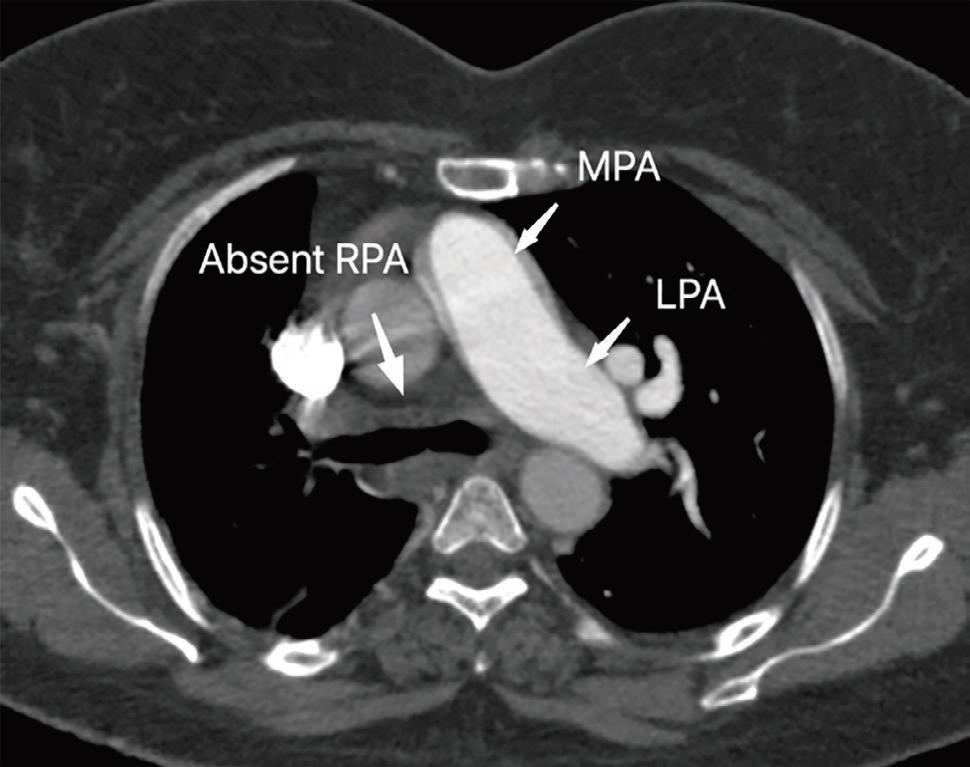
CT pulmonary angiogram phase in axial view shows complete absence of the right pulmonary artery (RPA). Main pulmonary artery (MPA) continues as left pulmonary artery (LPA).
